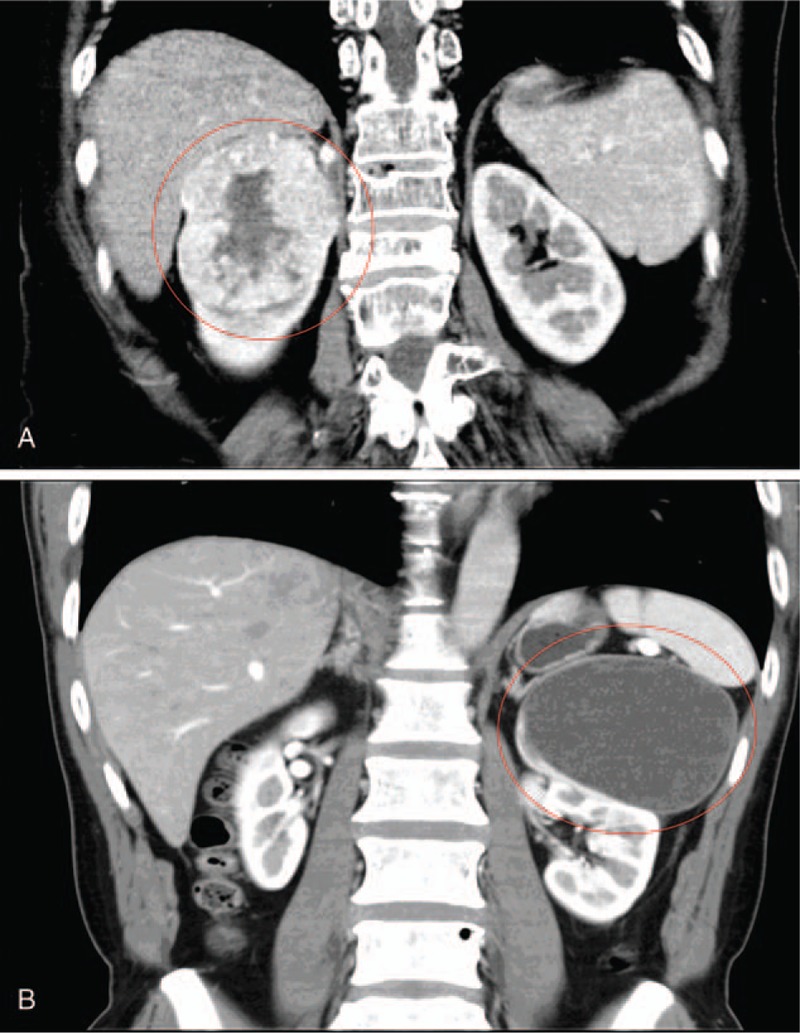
CT image of the patients’ abdominal organs. A, In Patient 1, CT revealed a well-defined solid tissue mass in the right kidney. B, In Patient 2, CT revealed a solid cystic mass in the left kidney. CT  = computed tomography.
